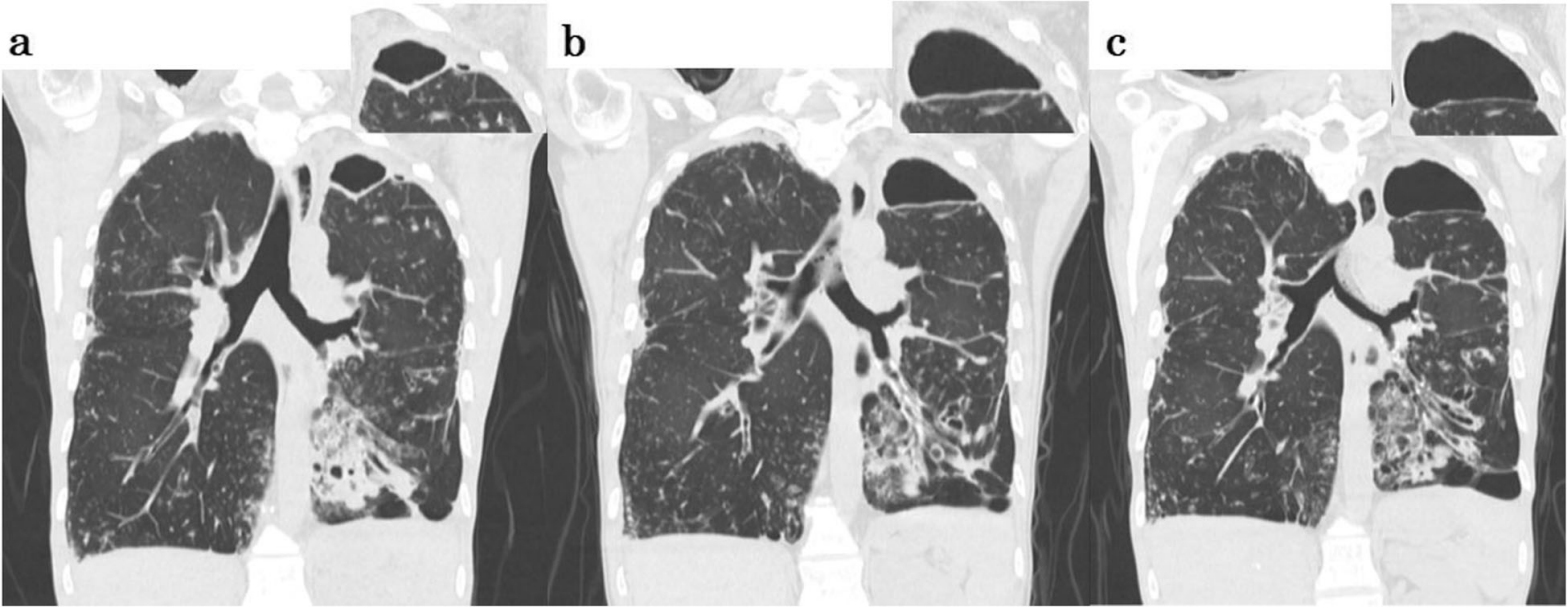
Comparison of the cavity lesion on coronal sections of high-resolution computed tomography. Images attached to the right shoulder are magnified views of cavity lesions. a A cavity lesion of the left lung apex was small at the first visit at our institution in 2014. b In 2017, the cavity lesion expanded and the wall thickened just before NTM treatment. c Repeat imaging in 2018, after NTM treatment, shows that the cavity wall is thinner
